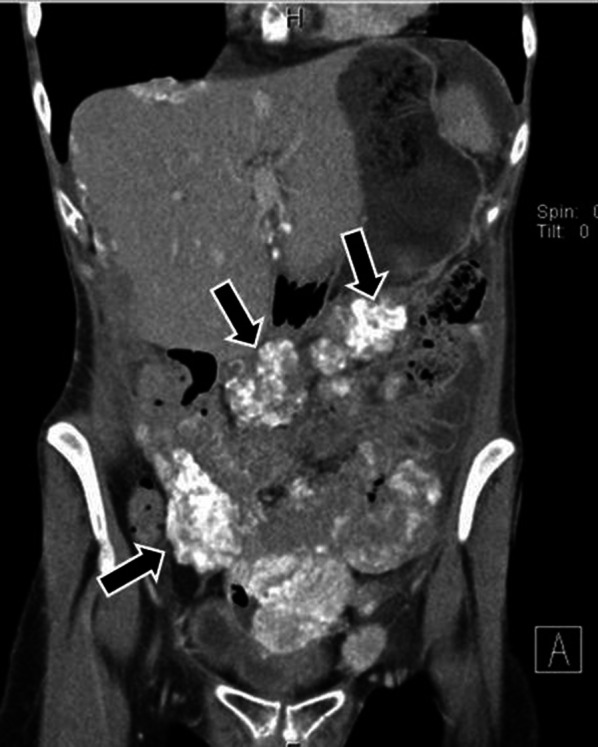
Patient with ovarian cancer and peritoneal carcinomatosis. Coronal reconstructed CT image shows multiple voluminous calcified implants in the peritoneum (black arrows)
